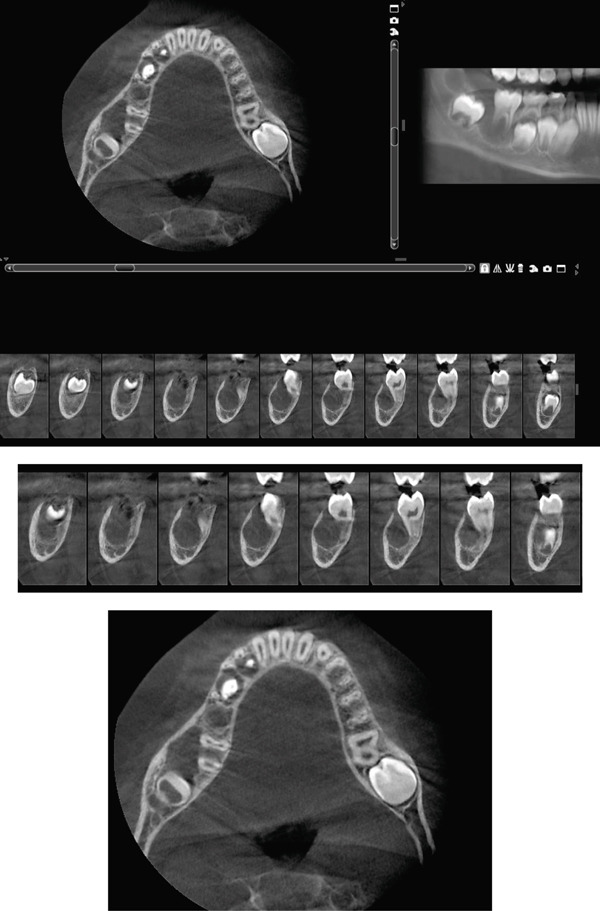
Radiography. CBCT, cone beam computed tomography.
